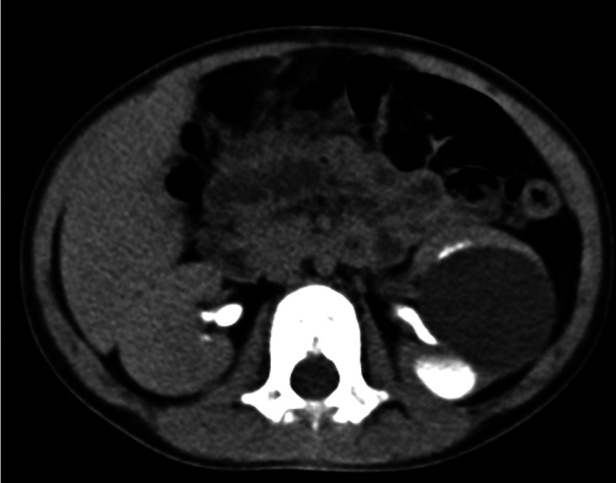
Image of an abdominal enhanced CT: unicystic tumor in the left kidney with a clear boundary but without contrast filling. There was contrast filling in the renal pelvis.
